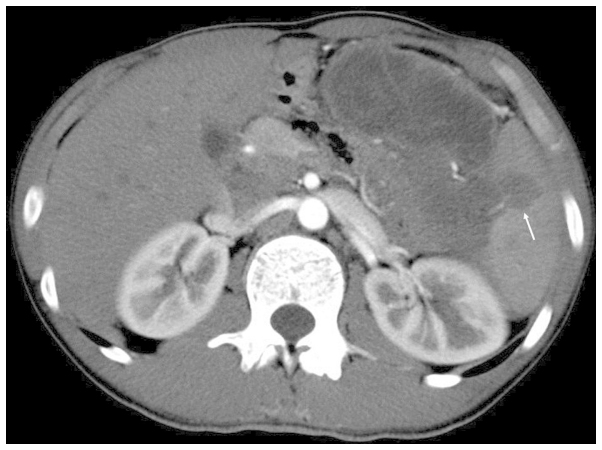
Contrast-enhanced computed tomography scan revealed that the tumor had invaded the spleen (shown by the arrow).
